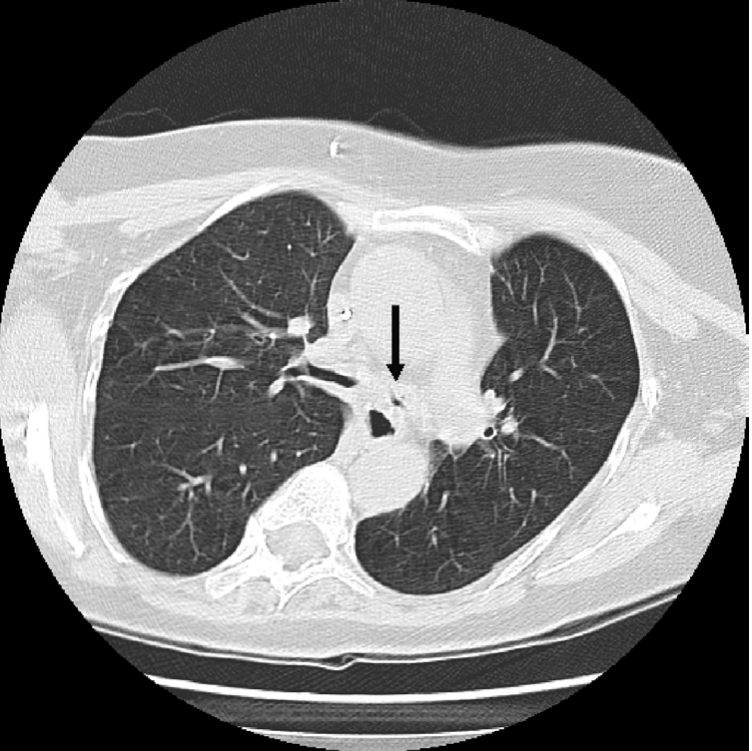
A CT scan section of case 2, showing severe stenosis of left main bronchus.
